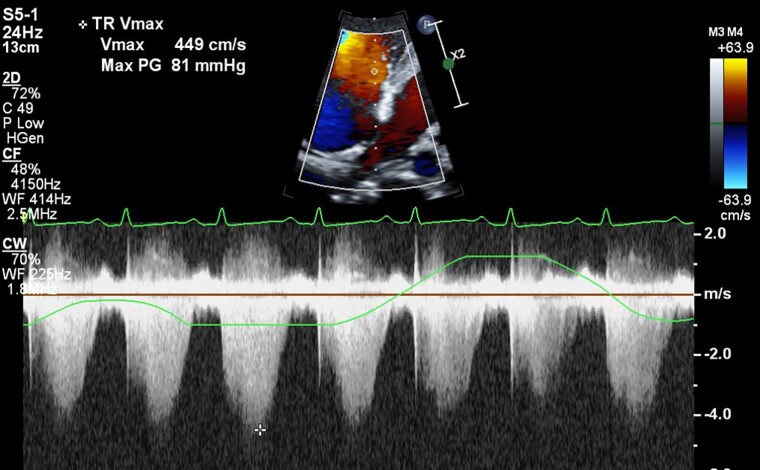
Transthoracic echocardiography showing continuous-wave Doppler across tricuspid valve showing a peak gradient of 81 mmHg.
